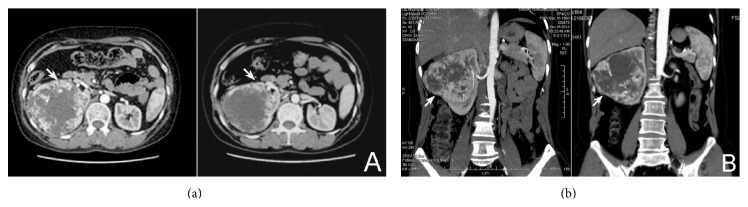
Transverse (a) and coronal (b) CT scans of a patient before (left) and after (right) sorafenib treatment. Arrows indicated the renal tumor.
